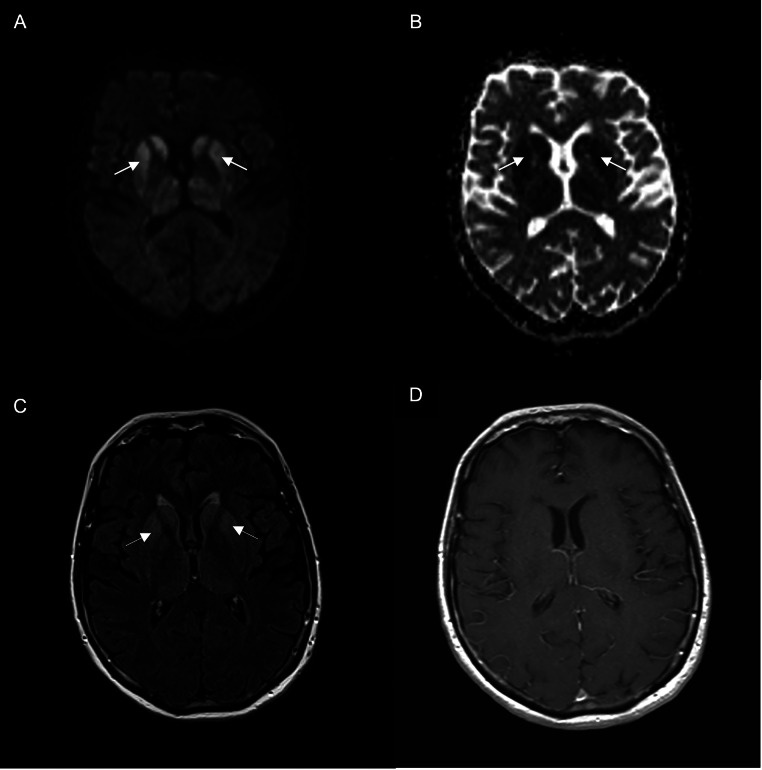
Axial DWI (A) and ADC (B) showed symmetric restricted diffusion in the bilateral caudate, putamen and thalami (thick arrows). Axial FLAIR (C) showed corresponding FLAIR hyperintensity (thin arrows). Axial contrast-enhanced T1 weighted sequence (D) showed no contrast enhancement.
